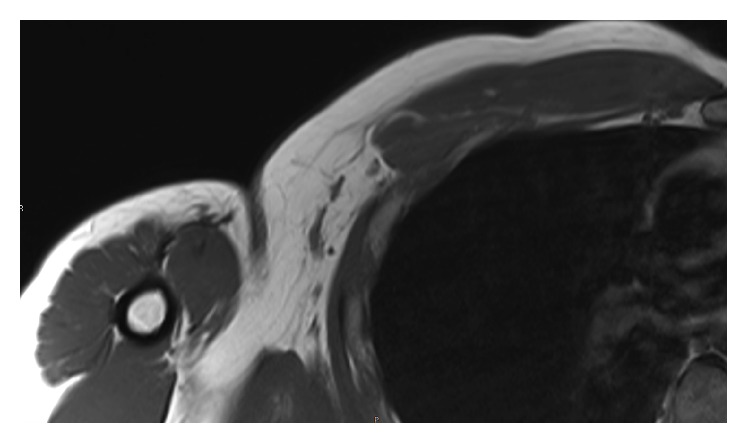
MRI axial T1 showing full-thickness PM tear at the humeral tendon-bone junction.
